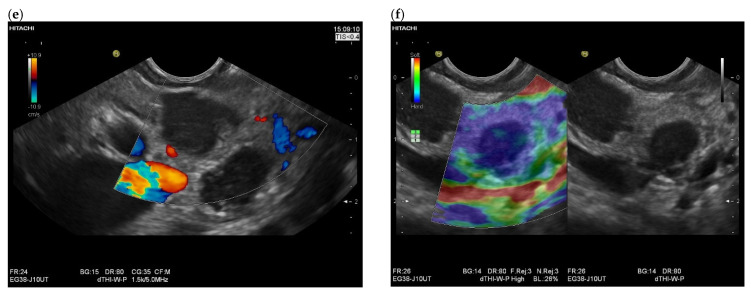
Intrapancreatic metastases of malignant melanoma in a male, 75 years old. Peripheral lymph node increasing for diagnosis. No abdominal complaints. B-mode ultrasonography shows a highly hypoechoic or anechoic lesion in the pancreatic body (a). In duplex, no vessels, no aneurysm. In CEUS, with 1.2 mL SonoVue in arterial phase at 18 s iso- to slight hypoenhancement (arrow) (b). In venous phase, slow washout at 42 s (c) with heterogeneous pattern at 1.14 min (d). EUS revealed additional multiple small hypoechoic lesions that had escaped percutaneous sonography. The lesions were highly hypoechoic, almost anechoic, smoothly confined, without evidence of vessels in the EUS duplex (e). Lesions were stiffer on strain elastography in EUS (f). The diagnosis of metastatic malignant melanoma was confirmed percutaneously, ultrasound-guided from the lymph nodes and by EUS-FNP from the pancreas.
